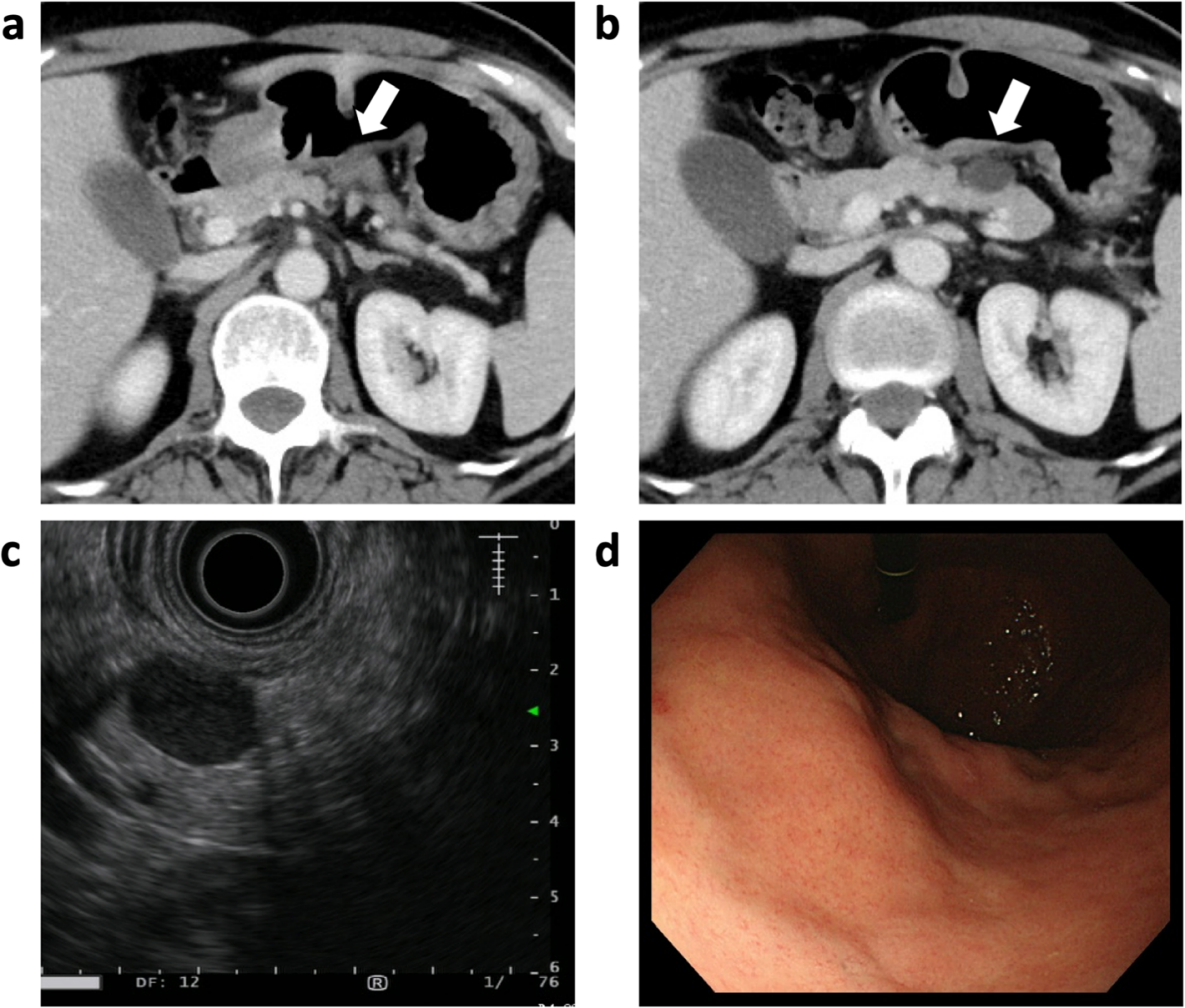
Preoperative images. a, b CT image showing a well-defined round, 20-mm cystic mass with a rim of soft tissue in the gastro-pancreatic region. The tumor is dense, adjacent to the gastric wall and pancreatic parenchyma. Slight enhancement of the solid component is seen after intravenous administration of contrast material. Arrows indicate the tumor. c Endoscopic ultrasound image showing a well-defined cystic mass. d Gastric endoscopy showed no abnormal findings of the gastric mucosa
